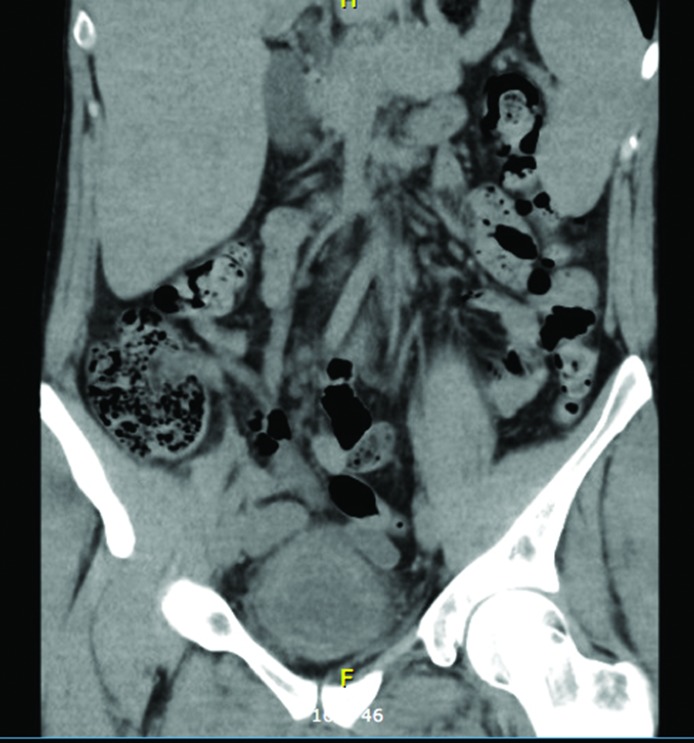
Coronal non-contrast CT KUB showing a markedly thick-walled bladder with peri-vesical stranding consistent with cystitis.
